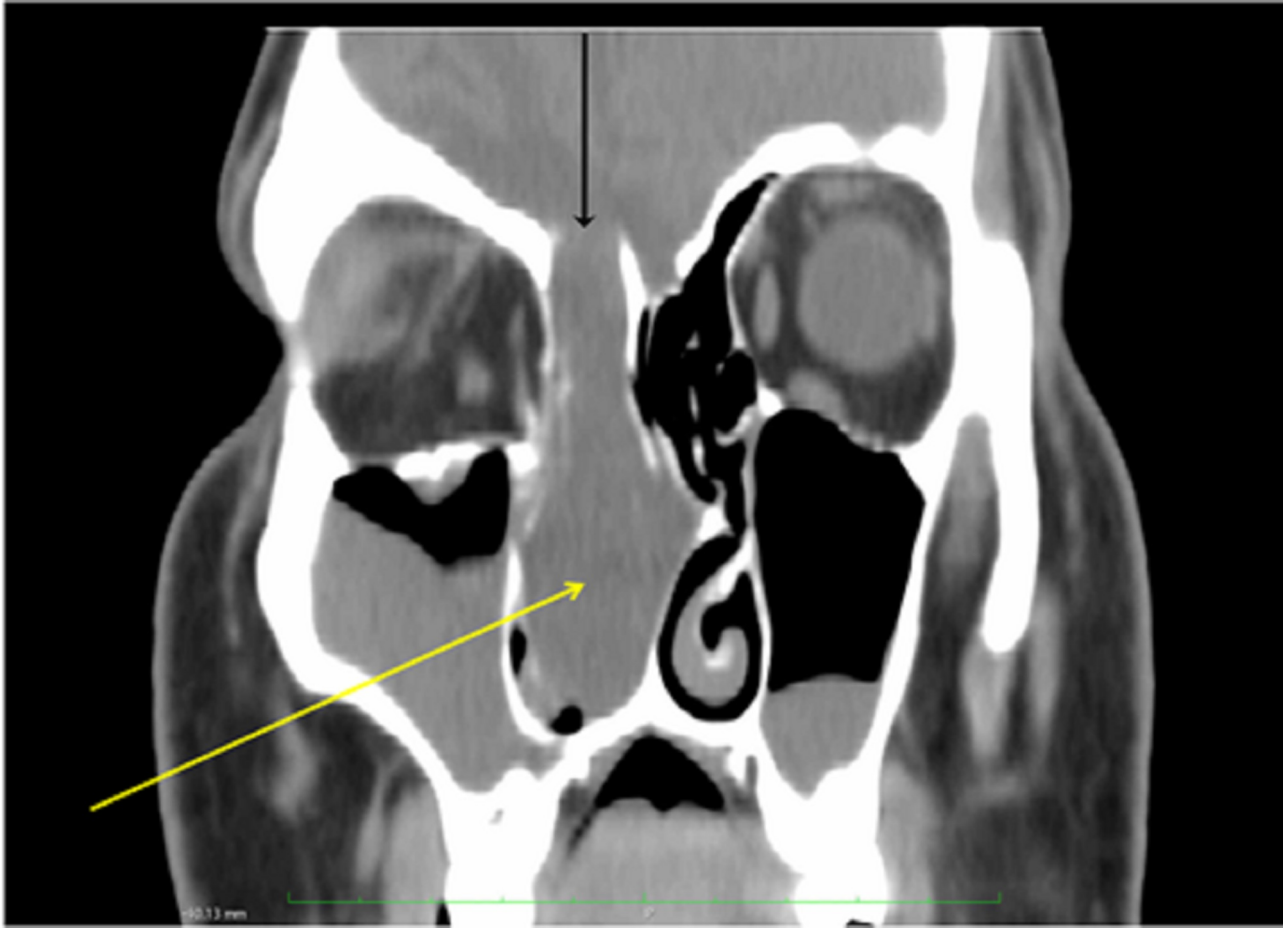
Preoperative craniofacial CT scan (coronal view) revealing a frontal bone gap around the top of the right ethmoid cells (black arrow) and a mass on the roof of the right nasal cavity. The rest of the right nasal cavity was filled with fluid with density similar to CSF (yellow arrow). CSF, cerebrospinal fluid
